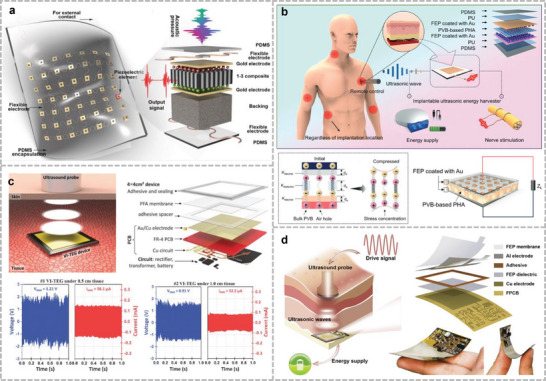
Ultrasound energy‐harvester for implantable device in vivo. a) Ultrasonic energy harvester arrays integrating a large number of piezoelectric active devices with multilayer flexible electrodes that produce continuous voltage and current outputs on planar and curved surfaces. Reproduced with permission.^[^
